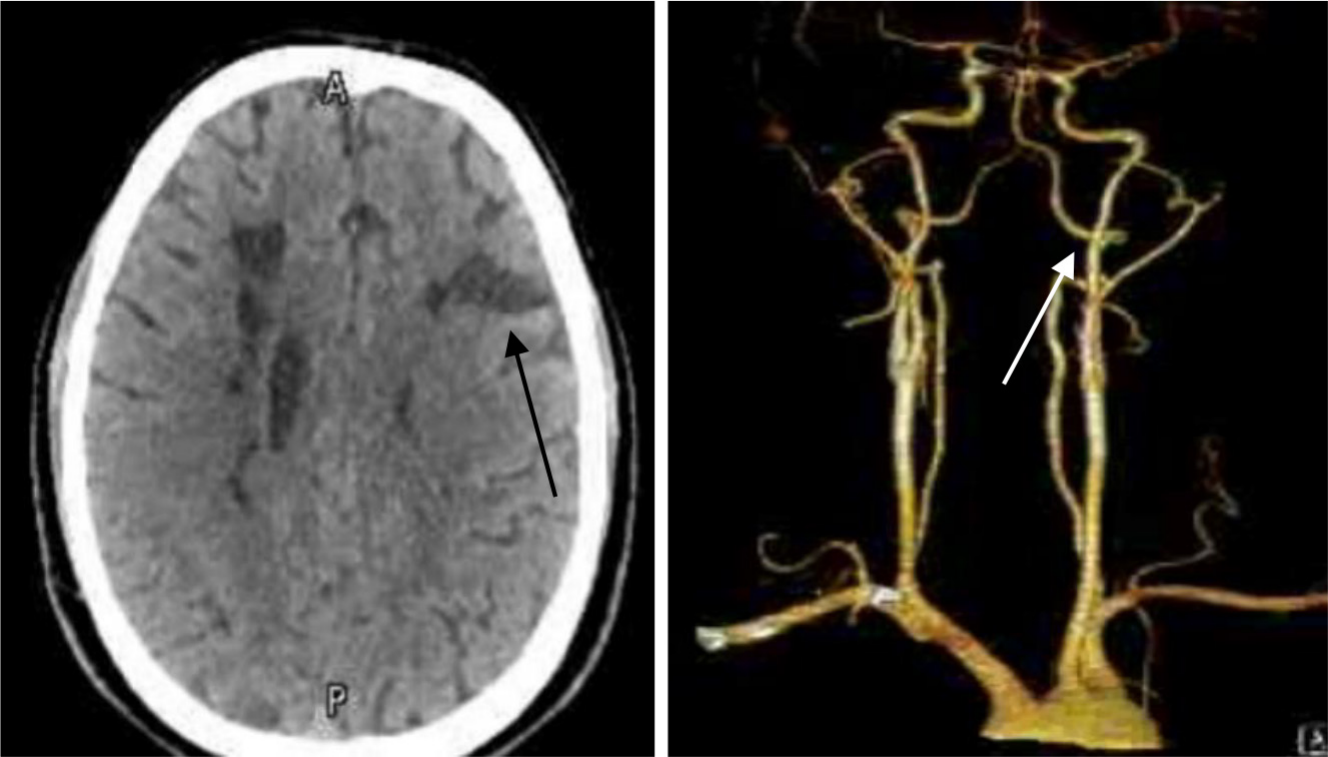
CT cerebral angiogram showed good filling of the bilateral common carotid arteries to the carotid bifurcation and the proximal cervical course of the internal carotid artery. The reduced caliber of the bilateral distal cervical course of the internal carotid arteries is seen with residual dissection, and focal outpouching of the right ICA is noted opposite C1 right lateral mass representing ICA pseudoaneurysm. The vertebral arteries bilaterally appear to be patent, with dominant left-sided noted.
